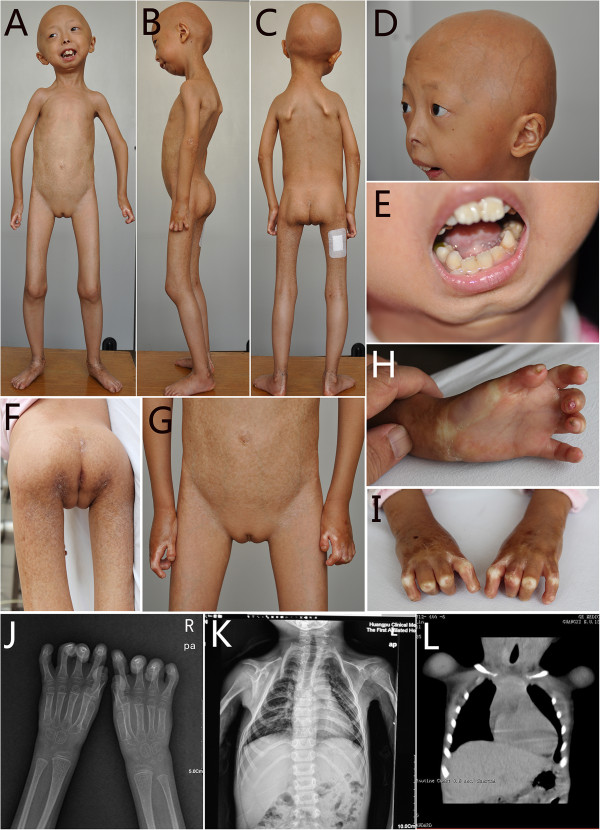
Patient 1 shows diffuse scalp hair loss, decreased eyebrow, prominent scalp veins, bulbous cheeks, tapered nasal tip, irregular teeth and lower jaw dental crowding, loss of subcutaneous fat, and mottled pigmentation and sclerodermatous changes over her trunk and lower limbs with protuberant abdomen and easily visible veins on abdomen (A-G), thickened skin on heels and around the ankles (A-C), severe contractures at the interphalangeal joints of the hands with flexion deformity of fingers (H, I). Lower skeletal density in upper limb with flexion deformity of fingers, and delayed bone age with absence of the lesser multangular bone, the great multangular bone, and the scaphoid bone (J). Pyriform thorax with absence of the clavicles, and absence of posterior parts of the 2^nd^ and 3^rd^ ribs on both sides, and thin posterior parts of the 4^th^ to 6^th^ ribs on left side and the 4^th^ on right (K). Normal clavicle images on the prior chest scan of computed tomography in 2009 (L).
